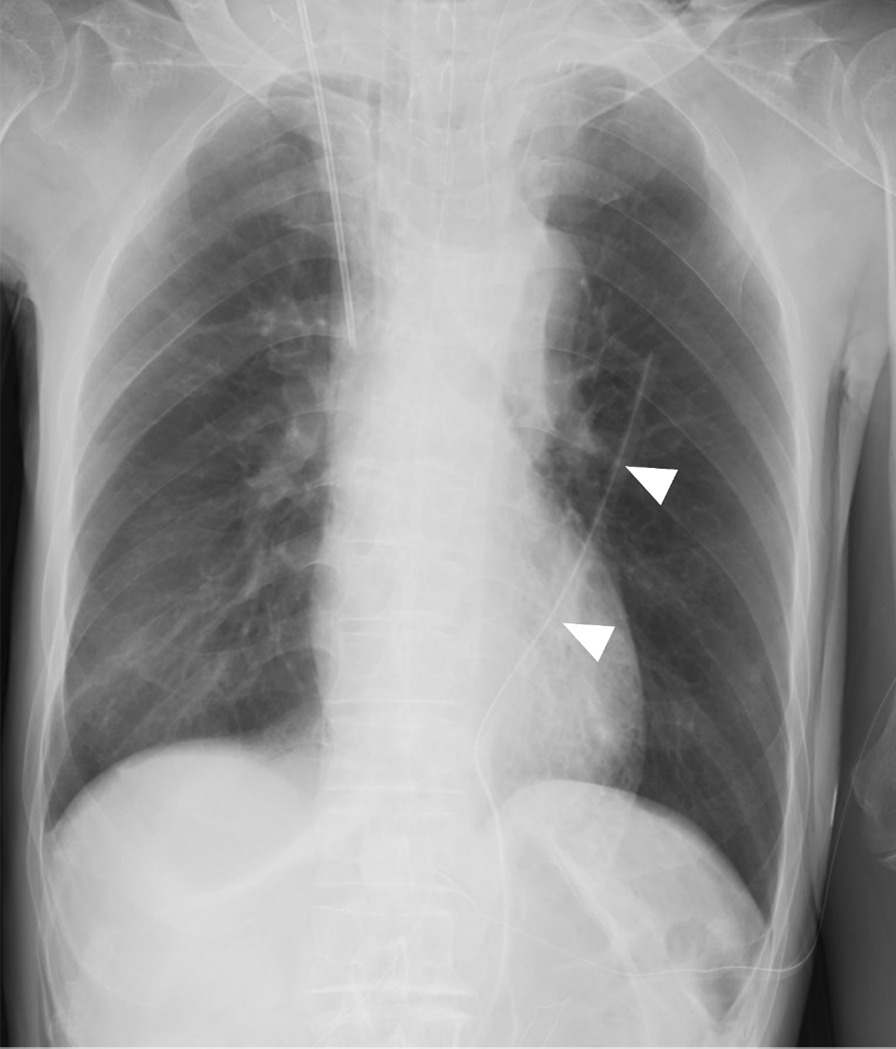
The postoperative chest radiography. Arrowhead, transhiatal left pleural drainage tube
